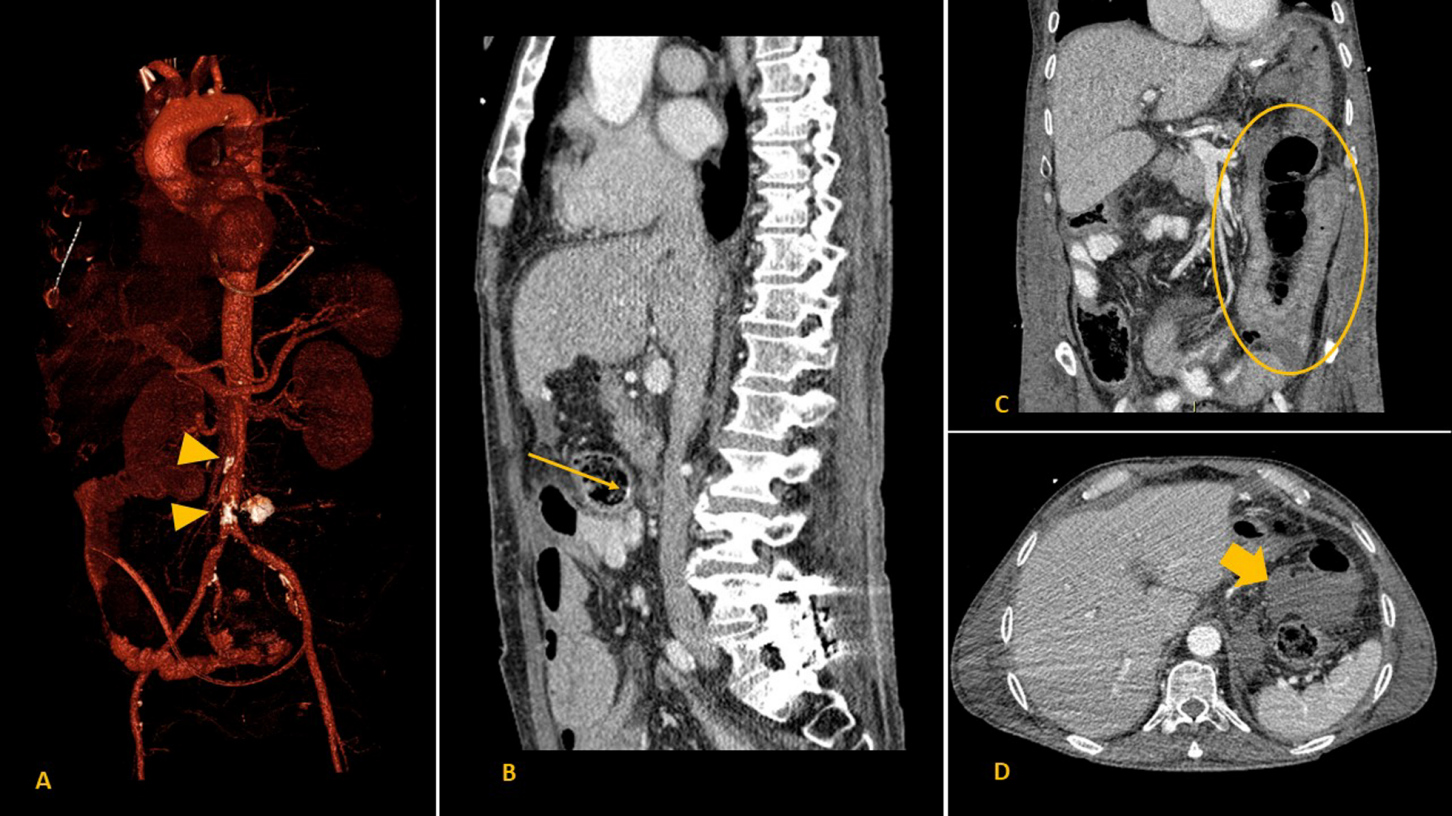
A–D Contrast-enhanced computed tomography (CECT) of the patient. A Three-dimensional (3D) vascular reconstruction that shows multiple atherosclerotic calcifications (triangles) but no severe stenosis in the main abdominal branches of the aorta. The CECT also displayed permeability of all veins. B Sagittal view of CECT, showing nonspecific linear hyperdensities of the colonic wall that could be related to incipient calcifications (arrow). C Coronal view of CECT, showing features of small bowel ischemia affecting the jejunum (circle), which led to a diagnosis of nonocclusive mesenteric ischemia. No ischemic changes in the colon were identified. D Axial view of CECT, showing that a moderate amount of intraperitoneal fluid was also present (thick arrow)
